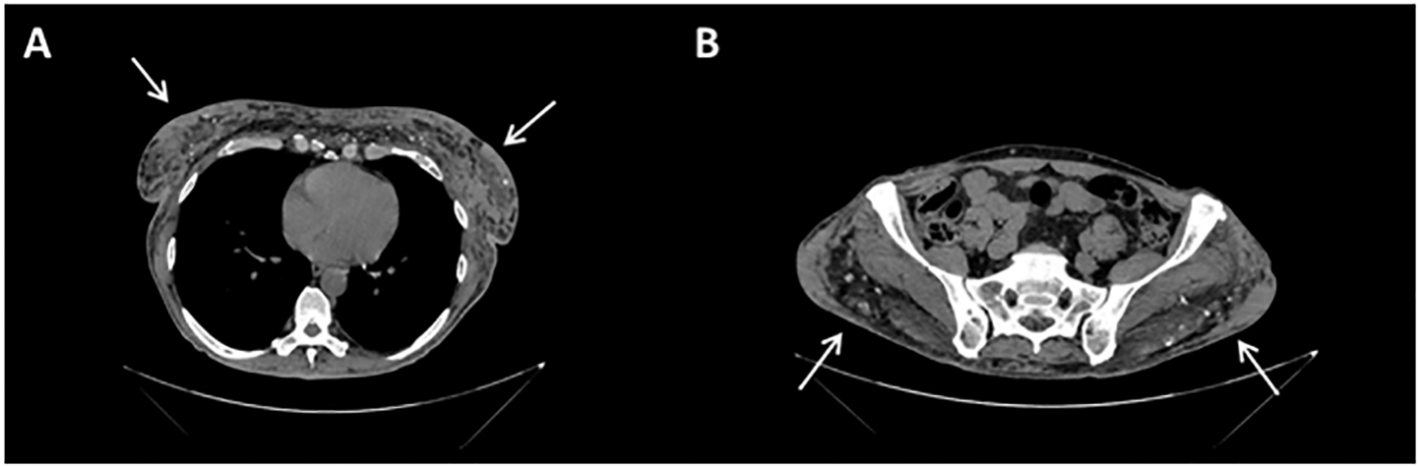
Contrast-enhanced CT scan sections: at mammary (A) and gluteal (B) level, images are compatible with patient history of silicone injections, in the form of soft-tissue densities with surrounding fat stranding (arrows) and peripheral calcifications.
